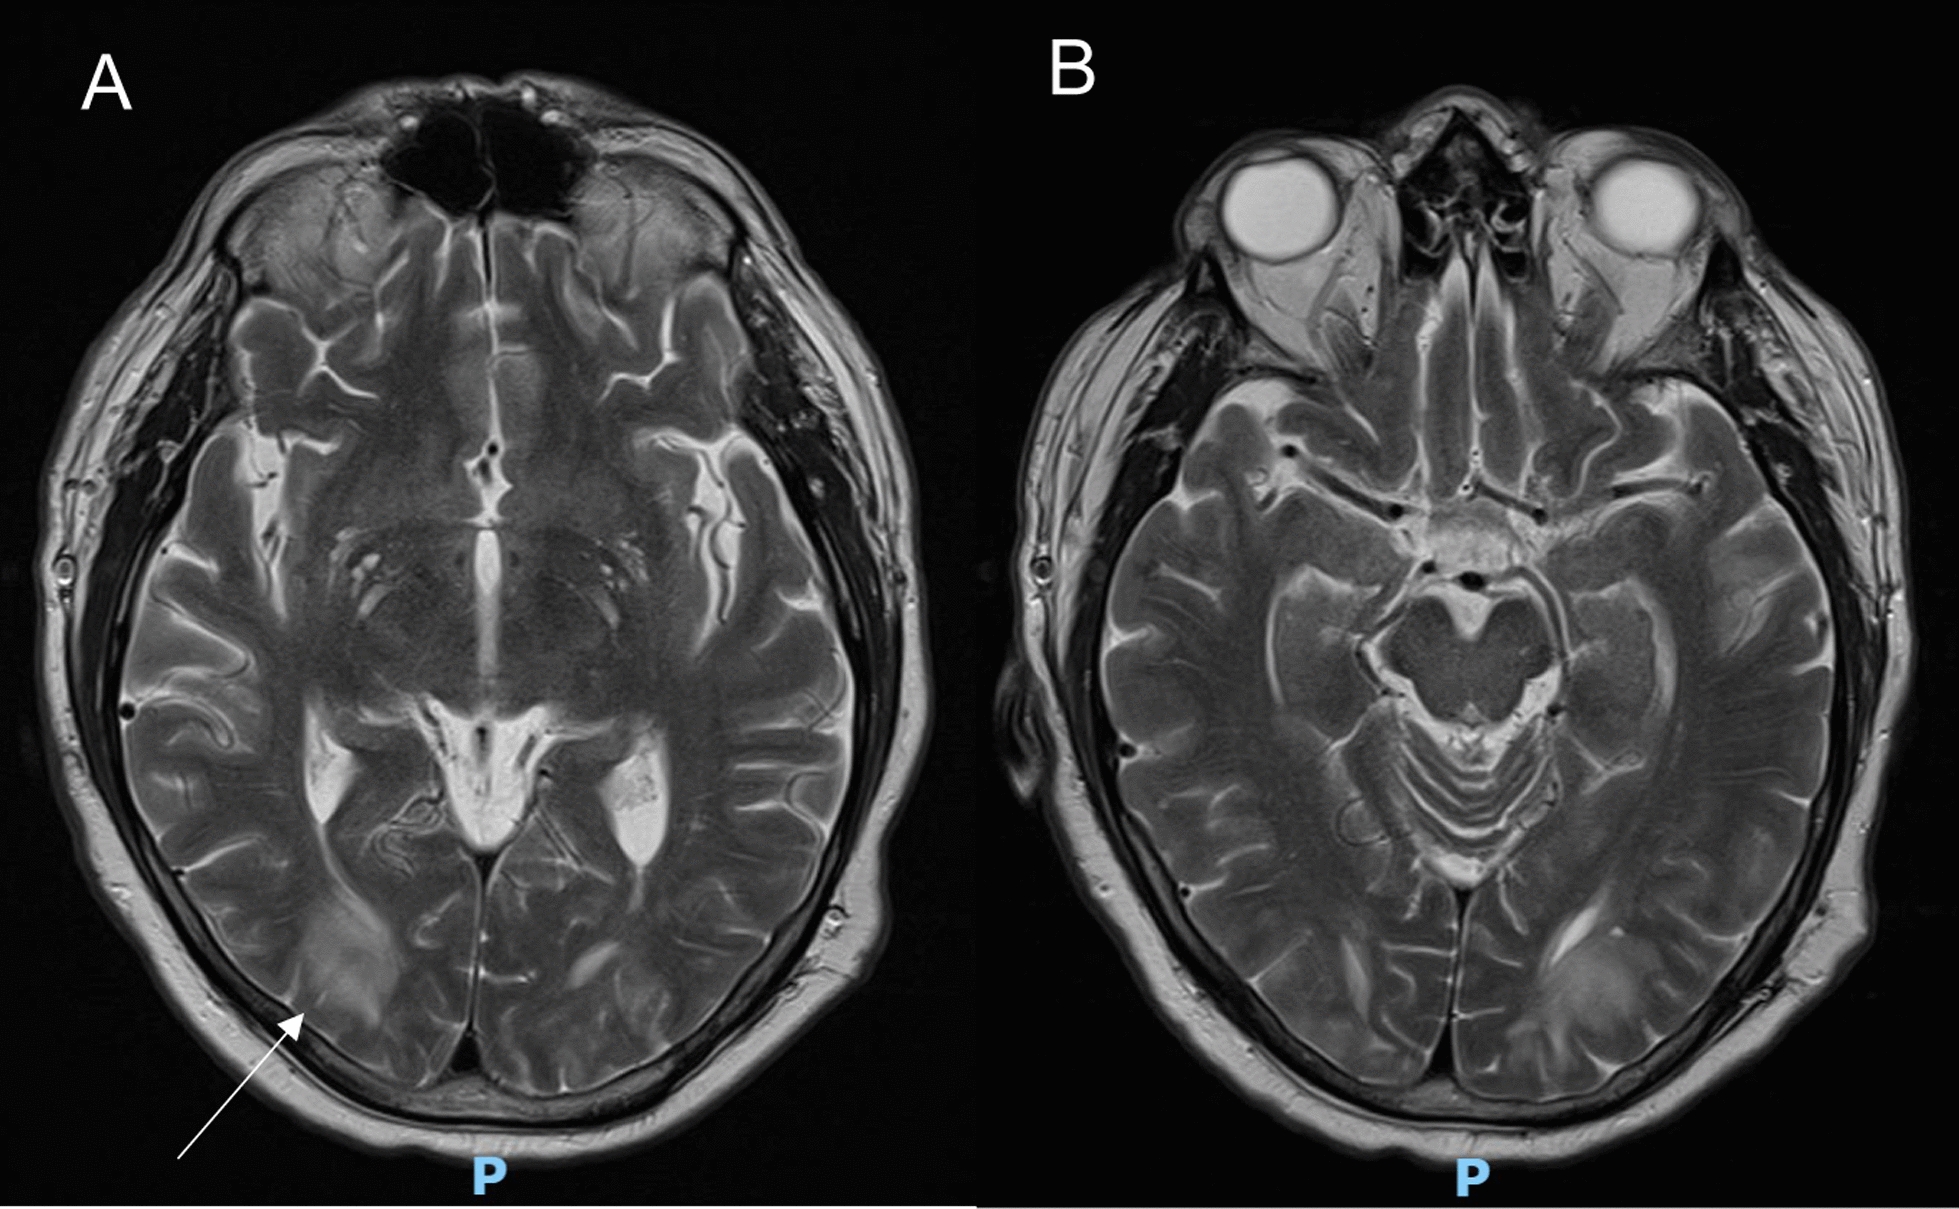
Coronal T2-weighted fluid-attenuated inversion recovery magnetic resonance imaging of the brain showing hyperintensity and mild volume expansion within deep and subcortical white matter of both posterior occipital lobes. A Hyperintense lesion in right occipital (white arrow). B Hyperintense lesion in left occipital (white arrow)
